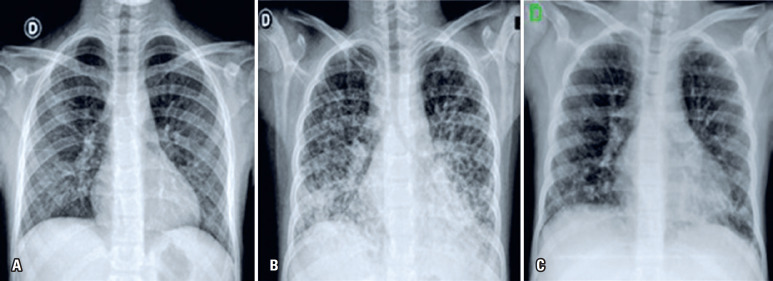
Chest X-rays of patients at baseline (A), 4 hours after intravenous immunoglobulin infusion (B), and 3 months later (C)
